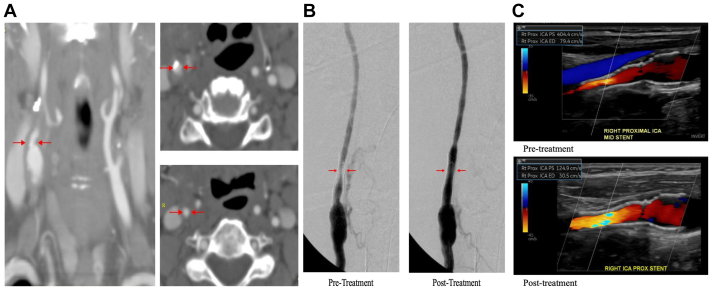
(A) Computed tomography angiogram of the neck before transcarotid artery revascularization (TCAR). Red arrows indicate area of right internal carotid artery (ICA) restenosis. (B) Intraoperative angiogram of right carotid artery before and after drug-coated balloon (DCB) angioplasty. Red arrows indicate area of restenosis. (C) Preoperative and postoperative duplex ultrasound examination of the right ICA. *PS*, peak systolic velocity; *Rt*, right.
